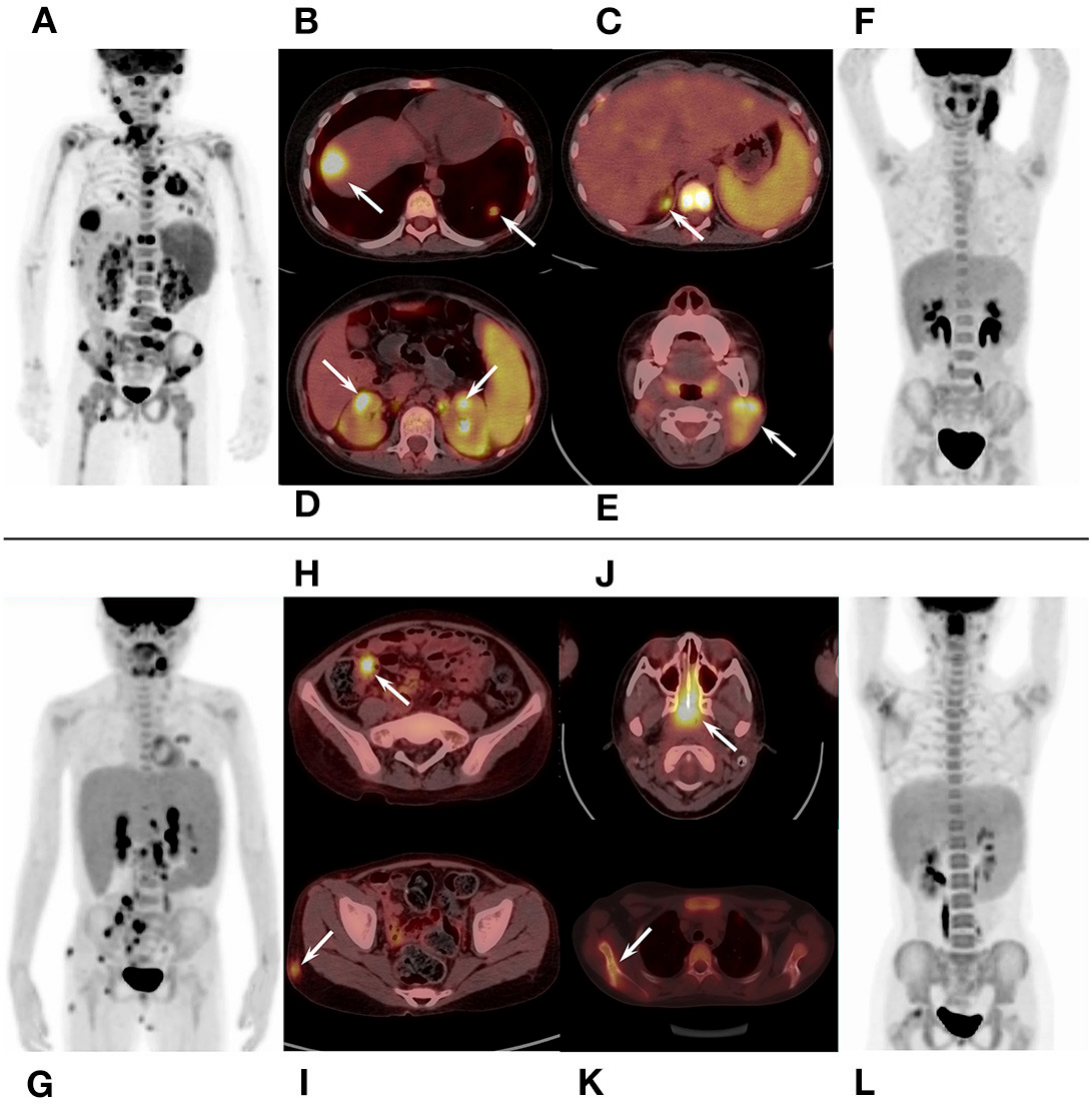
^18^F-FDG PET/CT findings of M-HLH. (A–D) A 6-year-old boy with HL, had multiple enlarged hypermetabolic lymph nodes and extronodal hypermetabolic lesions in multiple organs (nasal mucosa, liver, lung, adrenal gland, kidney, and bone marrow). (E,F) A 6-year-old boy with LPD grade 3, had local mass of fused lymph nodes in the neck; (G–I), a 10-year-old girl with NHL, had multiple enlarged hypermetabolic lymph nodes and extronodal hypermetabolic lesions in terminal ileum and skin. (J–L) A 10-year-old girl with NHL, had hypermetabolic lesions in nasopharynx and multiple lesions in scapula and femur. HLH, hemophagocytic lymphohistiocytosis; M-HLH, EBV-induced malignancy-associated HLH; LPD, lymphoproliferative disorders; HL, Hodgkin's Lymphoma; NHL, non-Hodgkin's lymphoma.
